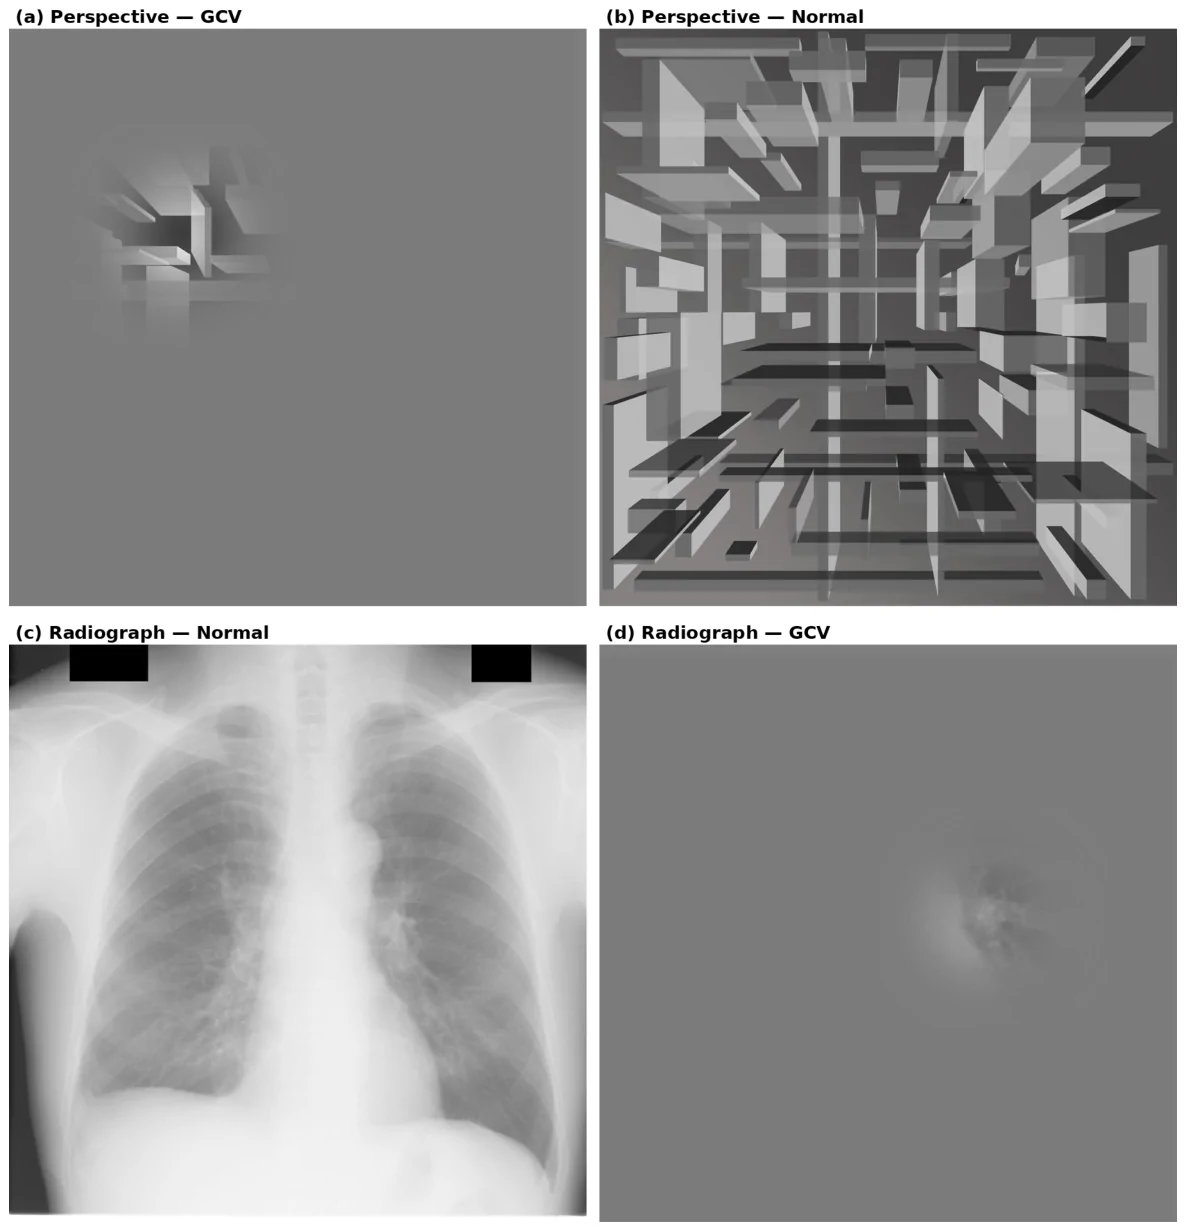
Example stimuli used in the experiment. (a) Zero-point perspective image under gaze-contingent viewing (GCV), showing the restricted viewing window. (b) The same perspective image under normal viewing. (c) Chest radiograph under normal viewing. (d) Chest radiograph under GCV.
